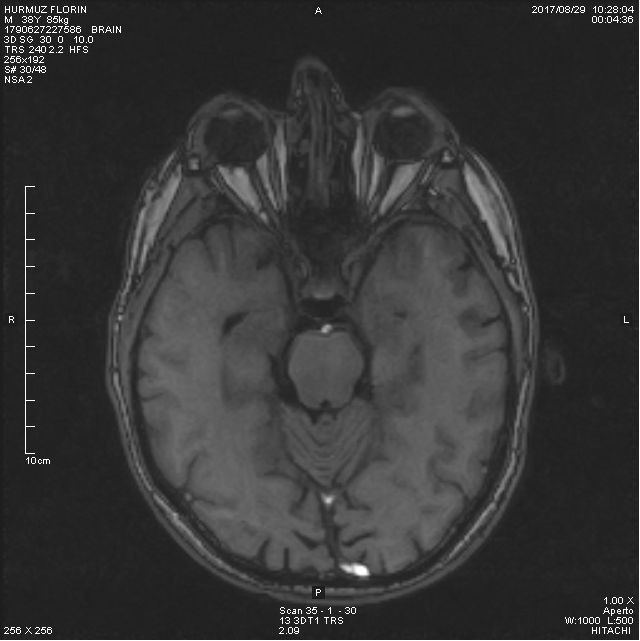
MRI in HF - The MRI in our patient showed that the mean values of optic nerve volumes were significantly lower
